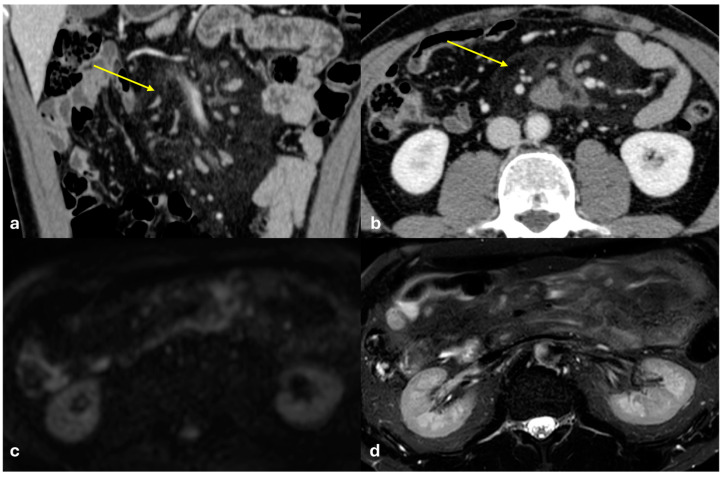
Mesenteric panniculitis. A middle-aged man, asymptomatic. CECT, made for blunt abdominal trauma, reveals hypertrophy of the mesenteric adipose tissue with soft tissue infiltration, a ring of normal fat surrounding the vessels and lymph nodes (fat ring sign) and a pseudocapsule ((a): coronal and (b): axial, arrows). MRI is made for further investigation: no significant restriction of diffusion is found (c) and mild hyperintensity is found on the T2-weighted image (d).
